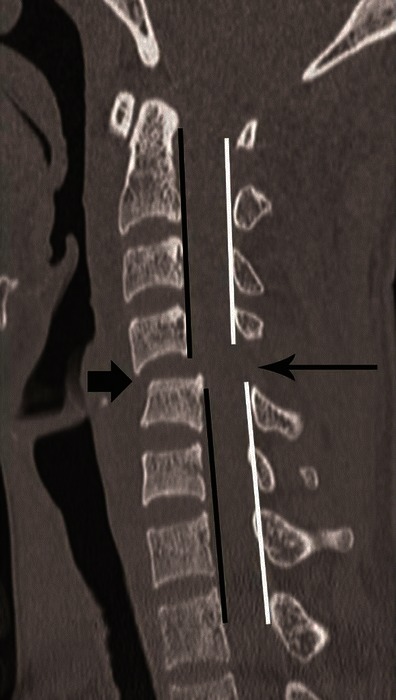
Hyperflexion distraction injury. Mid-sagittal CT image of the cervical spine shows focal kyphosis with posterior disc space widening at C4–C5 (*short arrow*). Mild anterolisthesis of C4 over C5 is seen suggested by the disrupted posterior vertebral line (*black lines*). The spinolaminar line is also disrupted with at C4–C5 with anterior displacement of C4 spinolaminar junction (*white lines*). Widening of the C4–C5 interlaminar/interspinous space (*long arrow*) is noted. Combination of this pattern of injury is highly suggestive of hyperflexion distraction injury and the facet joint should be carefully evaluated on lateral parasagittal images for possible distraction, subluxation and dislocation (shown in Fig. 13)
